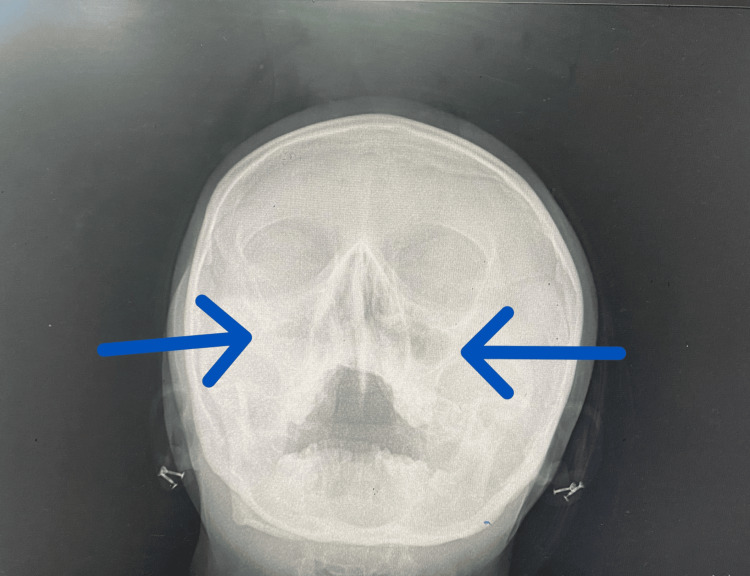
An X-ray of the paranasal sinuses demonstrates mucosal thickening and opacification, consistent with chronic sinusitis.
